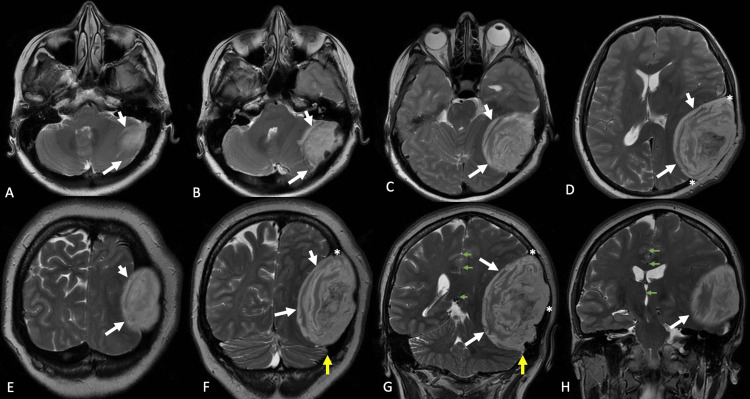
Preoperative axial (A-D) and coronal (E-H) T2 fast spin-echo MRI images demonstrate the lesion (white arrows) with a heterogeneous, partially laminated hyperintense signal and pronounced mass effect on the adjacent cerebral parenchyma. The asterisk indicates the bone defect, and green arrows indicate the midline shift.
